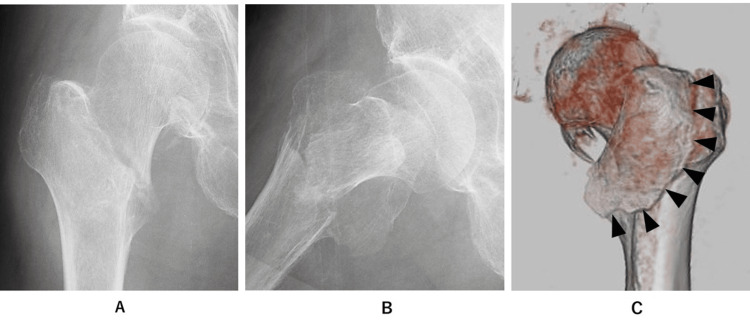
Case presentation Using two-dimensional plain radiographs (A: anterolateral view, and B: lateral view), the fracture was classified as Type A-1.3. However, CT scan images (C: 3D CT image) revealed a large-sized pertrochanteric fragment from the greater trochanter to the trochanteric crest, lesser trochanter (indicated in black arrowheads). Hence, the fracture was re-classified as Type A-2.2.
